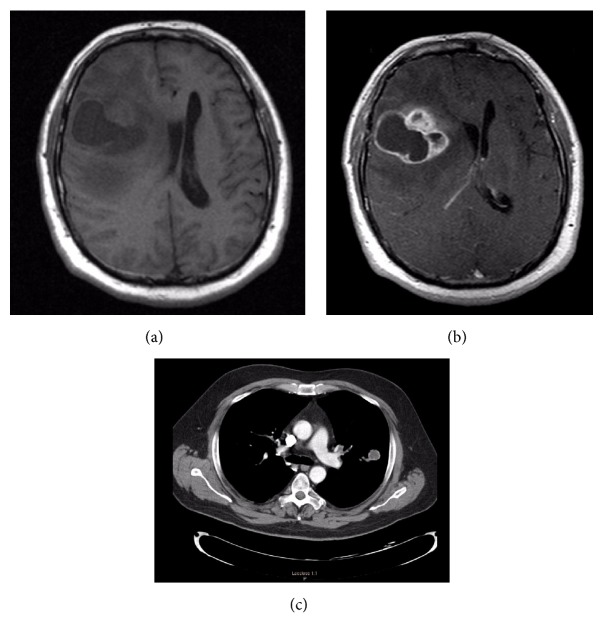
(a) and (b): pre- and postcontrast MRI demonstrating large right frontal ring-enhancing mass, October 2008. (c) Chest CT with mass in the left lung, May 2009.
